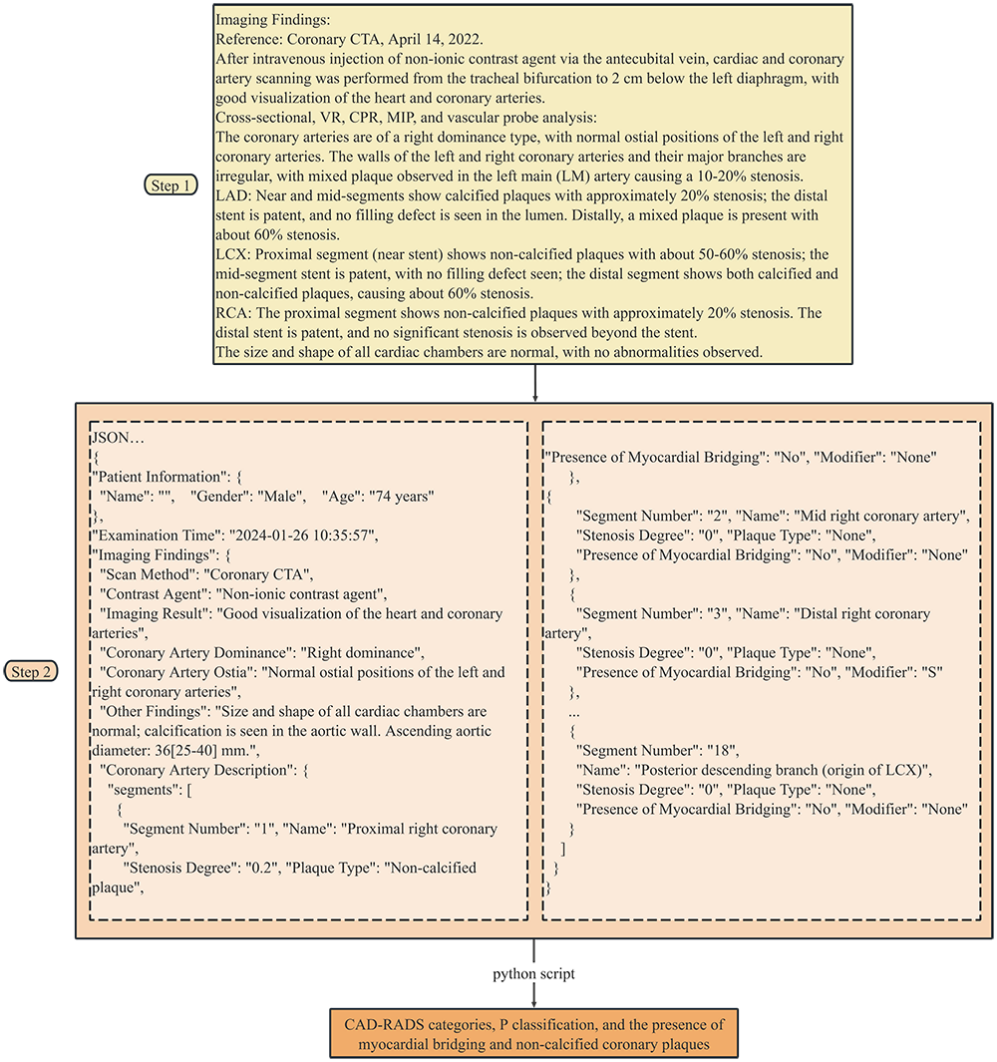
A representative example showing how GPT-4o processes and structures a coronary computed tomography angiography radiology report. The system uses a specialized prompt to transform the free-text report into a standardized JSON format, comprising 18 distinct sections (sections 1‐18, with intermediate sections abbreviated for brevity). The structured output is subsequently analyzed by a Python script to identify the Coronary Artery Reporting and Data System categories, P categories, myocardial bridge, and noncalcified plaque.
